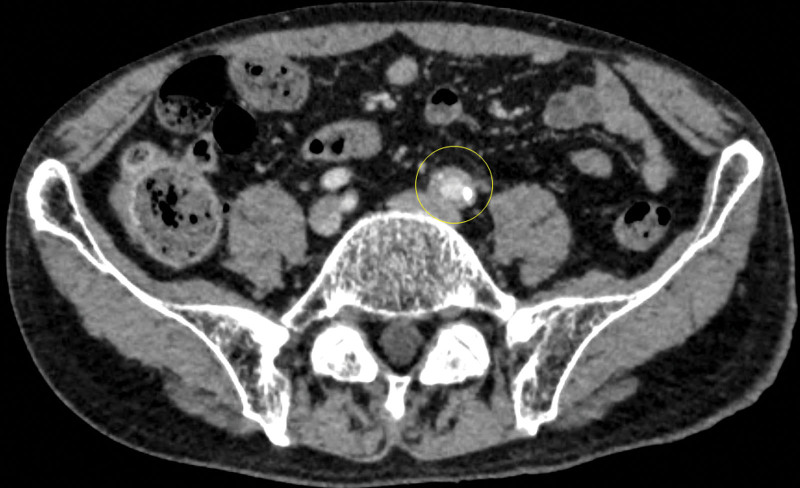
CT findings were suggestive of periaortitis of the abdominal aorta up to the common iliac artery (circle). CT = computed tomography.
